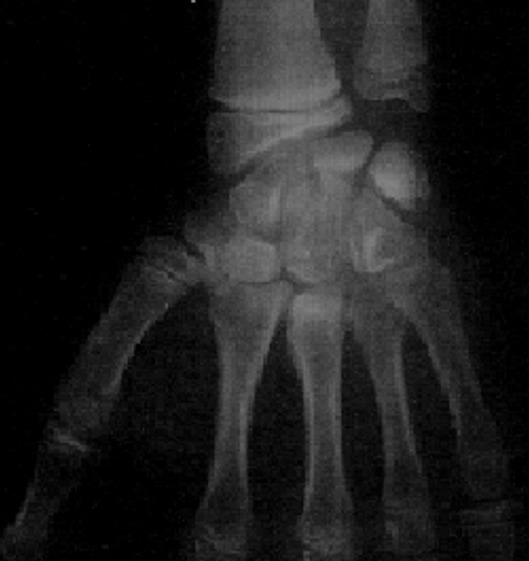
Face radiograph shows healing of the lunate
